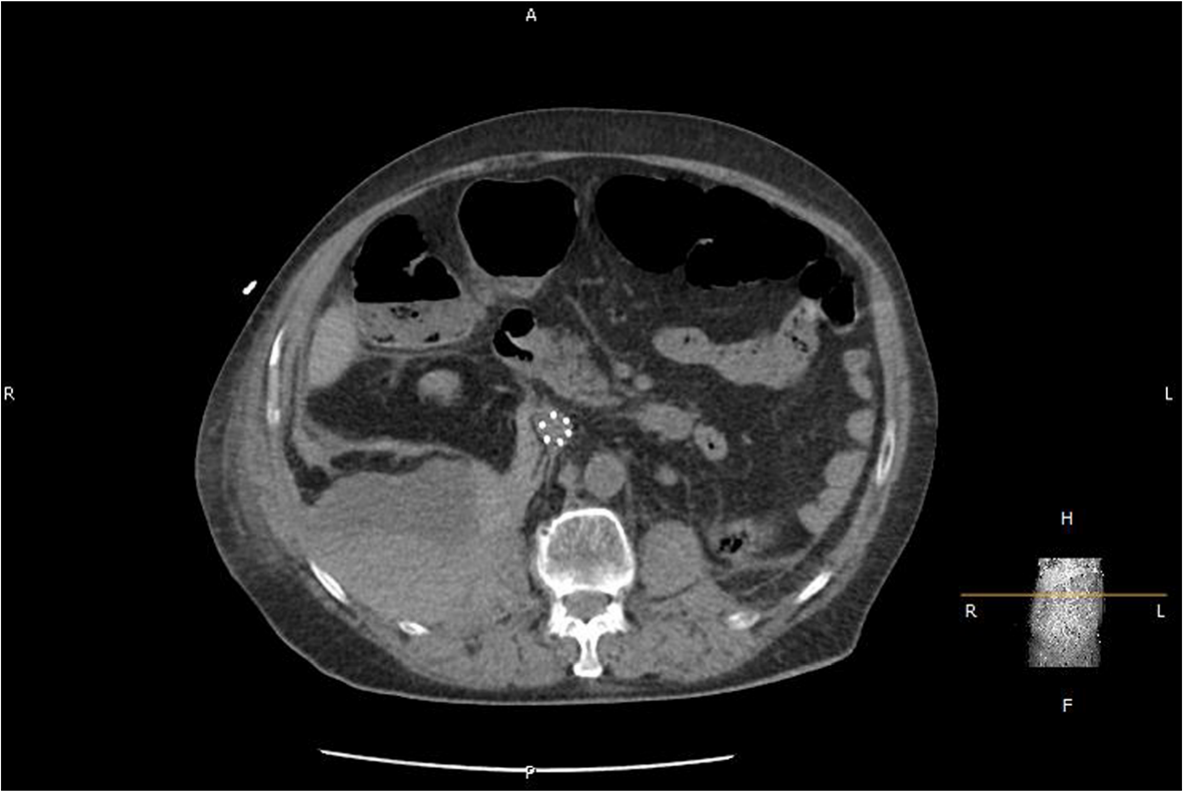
Repeat abdomen and pelvis computed tomography performed on hospital day 10. The implanted inferior vena cava filter can also be seen
